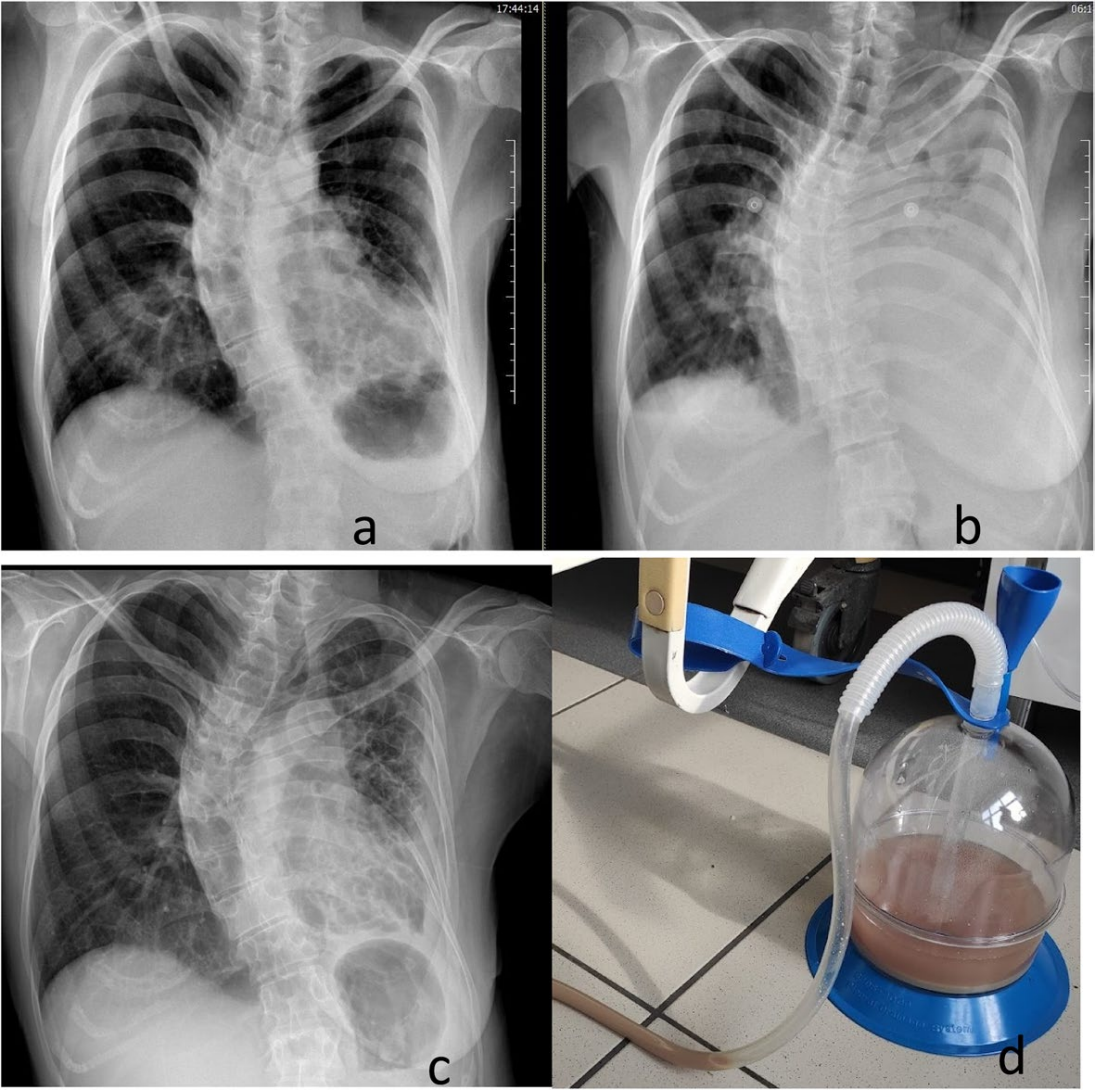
a Chest X-ray at hospitalization; b chest X-ray when empyema was detected; c chest X-ray at the second month of treatment; 1d Empyema drainage with chest tube
